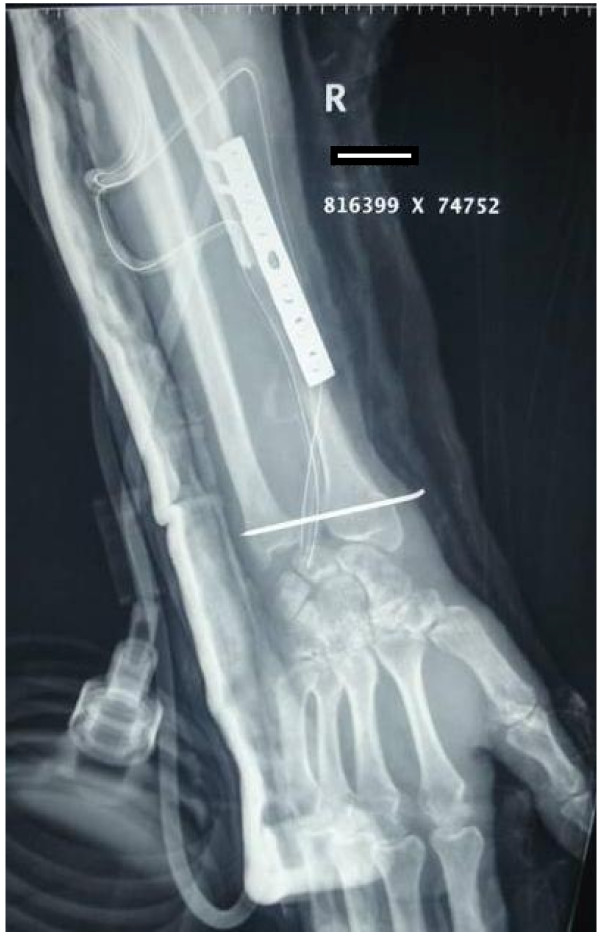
Immediate post operative radiograph of the same patient.
